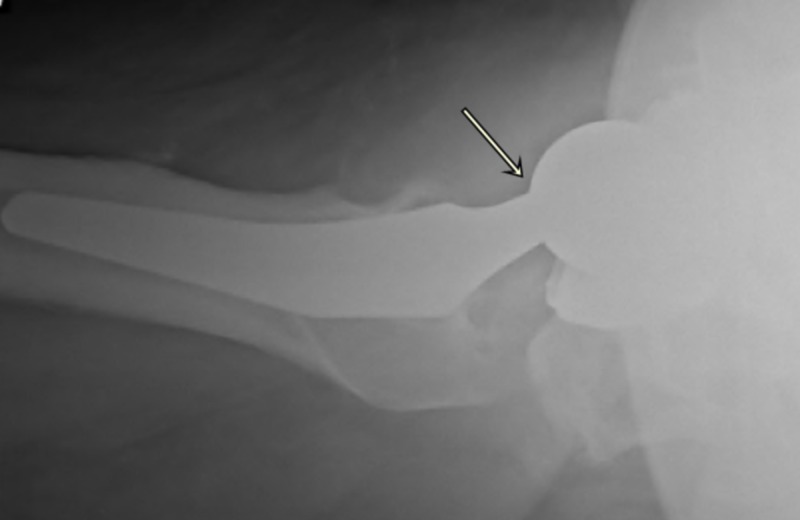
Lateral radiograph of the pelvis with head neck dissociation of the right hip implant
